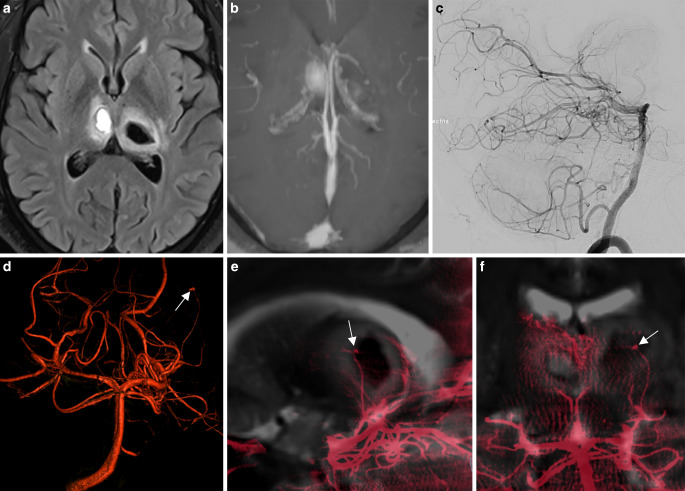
Ruptured thalamo-perforating branch micro-aneurysm. a Baseline magnetic resonance imaging (MRI) demonstrating a bithalamic hemorrhage. b No sign of central venous thrombosis. c Digital subtraction angiography (DSA) run of the posterior circulation in the lateral projection with normal findings. d Three-dimensional rotational angiography (3DRA) showing a small aneurysm (*white arrow*) in the course of a left thalamo-perforating branch. e,f 3DRA + MRI fusion clearly demonstrates the aneurysm located within the left thalamic hemorrhage as the bleeding source
